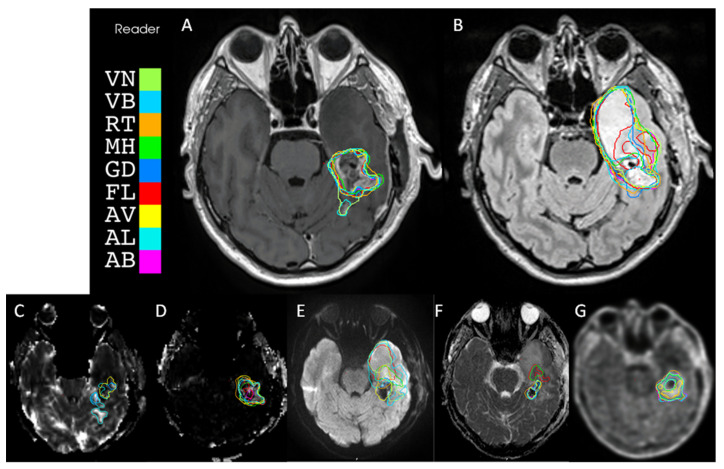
Volume target variability between readers for each sequence. (A). T1-weighted imaging (T1Gado). (B). T2-weighted fluid-attenuated inversion recovery (T2Flair). (C). Relative cerebral blood volume (rCBV) corrected for contrast leakage shows. (D). Permeability estimation map (k2). (E). Diffusion-weighted imaging (DWI b1000). (F). Apparent diffusion coefficient (ADC) map. (G). 18F-FET PET/CT.
